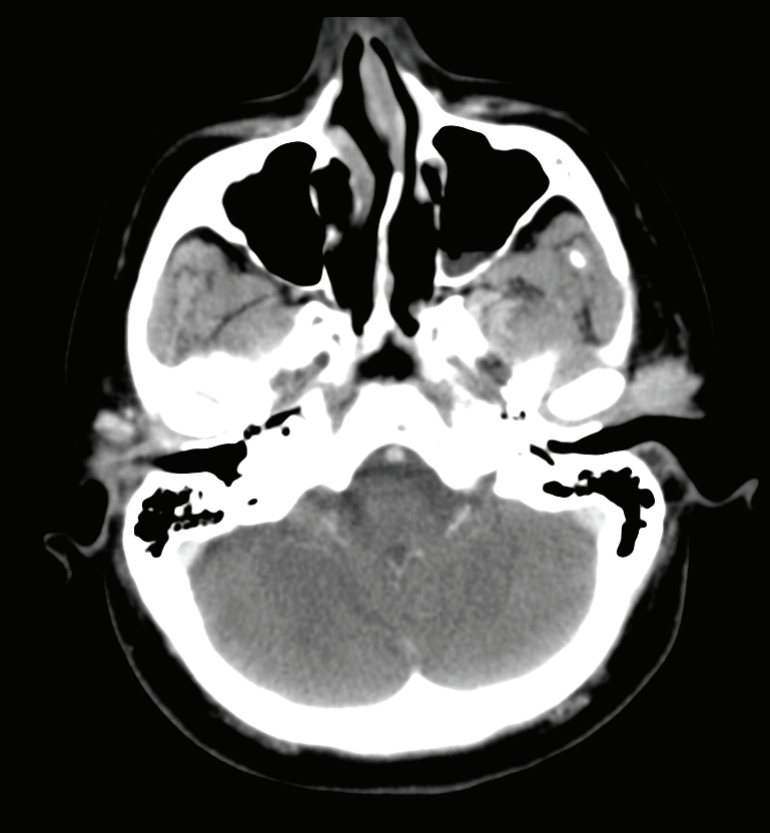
A contrast-enhanced axial CT image shows an enhancing, lobulated mass in the left preauricular region.
